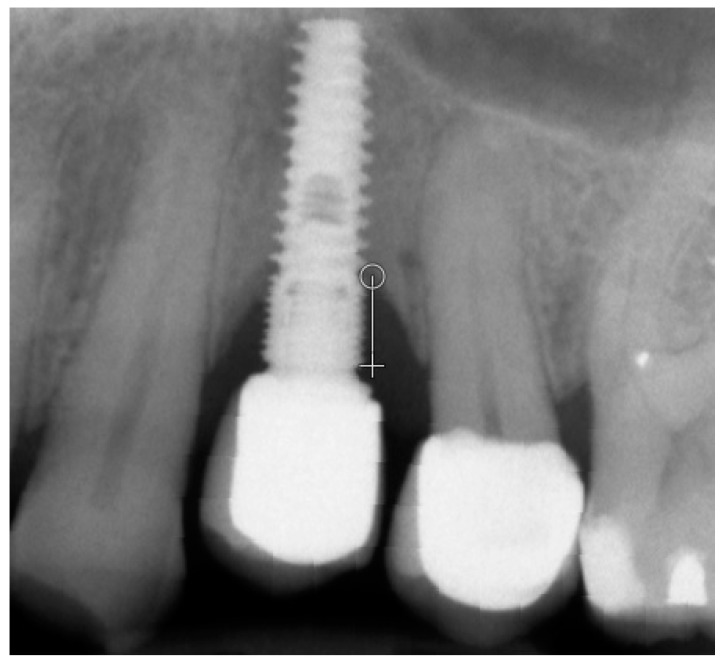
Measuring of marginal bone loss on the radiographic images 5 years after the functional loading. White line indicates the implant platform to the bottom of the bone loss cavity.
